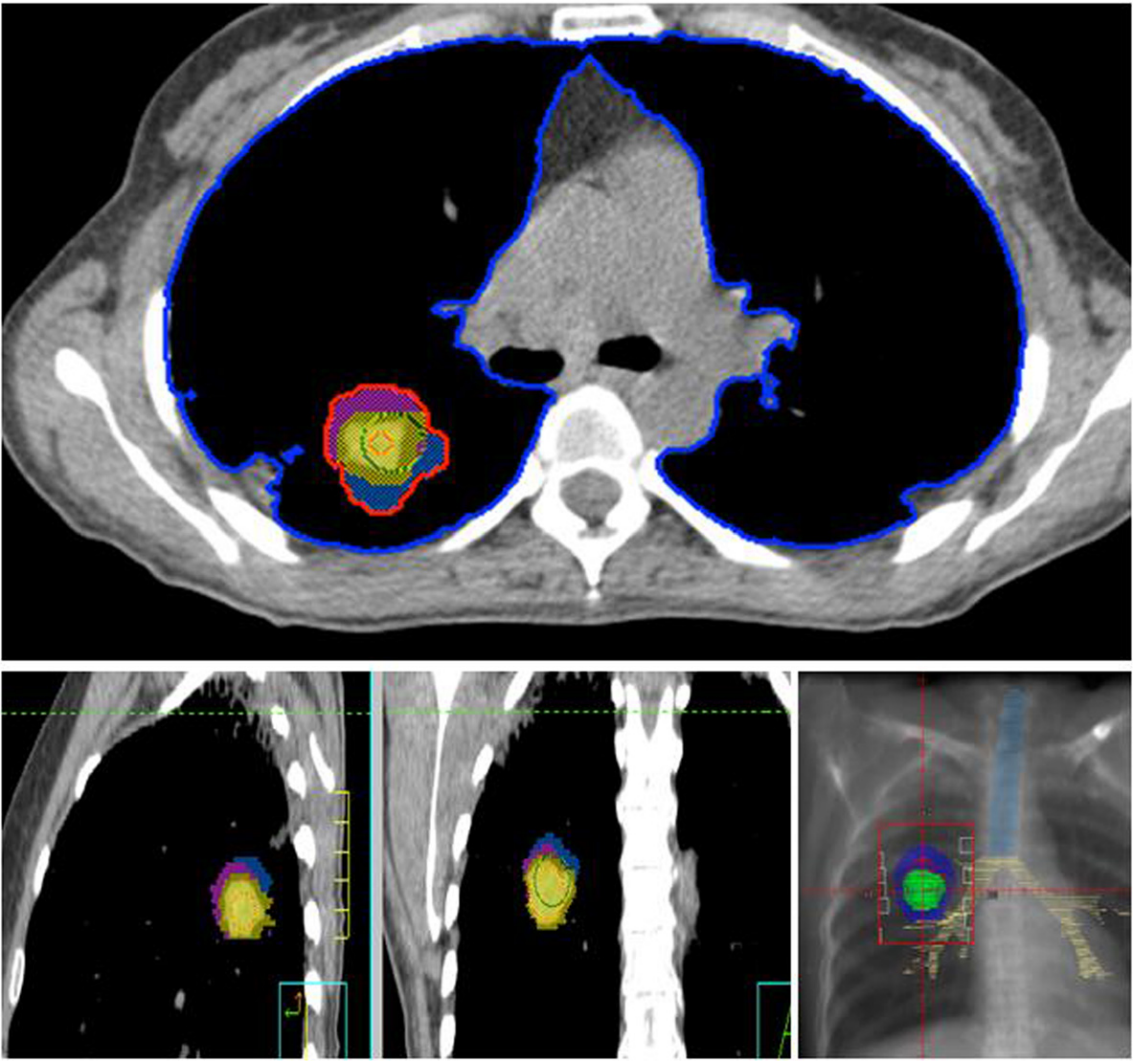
A combination set of three CT scans obtained during free breathing (pink), deep inspiration (yellow) and deep expiration (blue) were used to generate an internal target volume (ITV) that accounts for respiratory motion (red). The blue dots outline the lung structure and the red dots indicate the radiation target. Digital Reconstructed Radiography (DRR) show the trachea (blue) and bronchial tree (yellow) structure and the targets volumenes (the lung nodule (green), and the PTV (dark blue) included the ITV (red) with a symmetric 0.5 cm margin.
